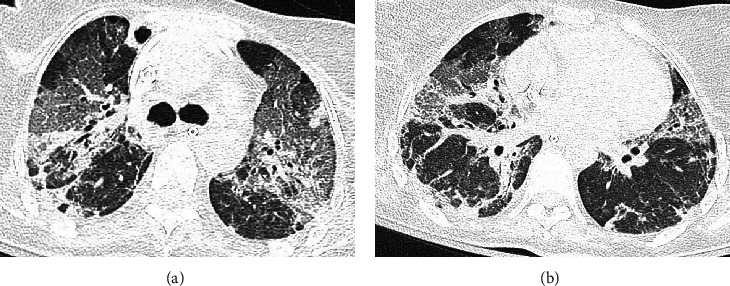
(a, b) CT images showing the switch to bilateral fibrosis of the lungs due to prolonged mechanical ventilation during the weaning trial from the venovenous ECMO.
